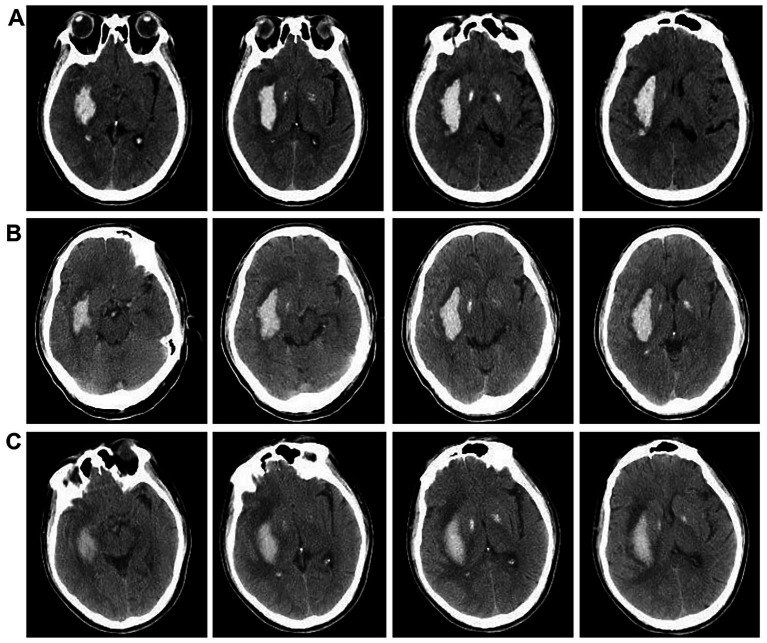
CT Comparison images before and after medication intervention treatment. (A) Brain CTA scan images of patients upon admission. (B) Brain CT scans of patients after 3 days post-admission. (C) Brain CT scans of patients after 7 days post-admission.
